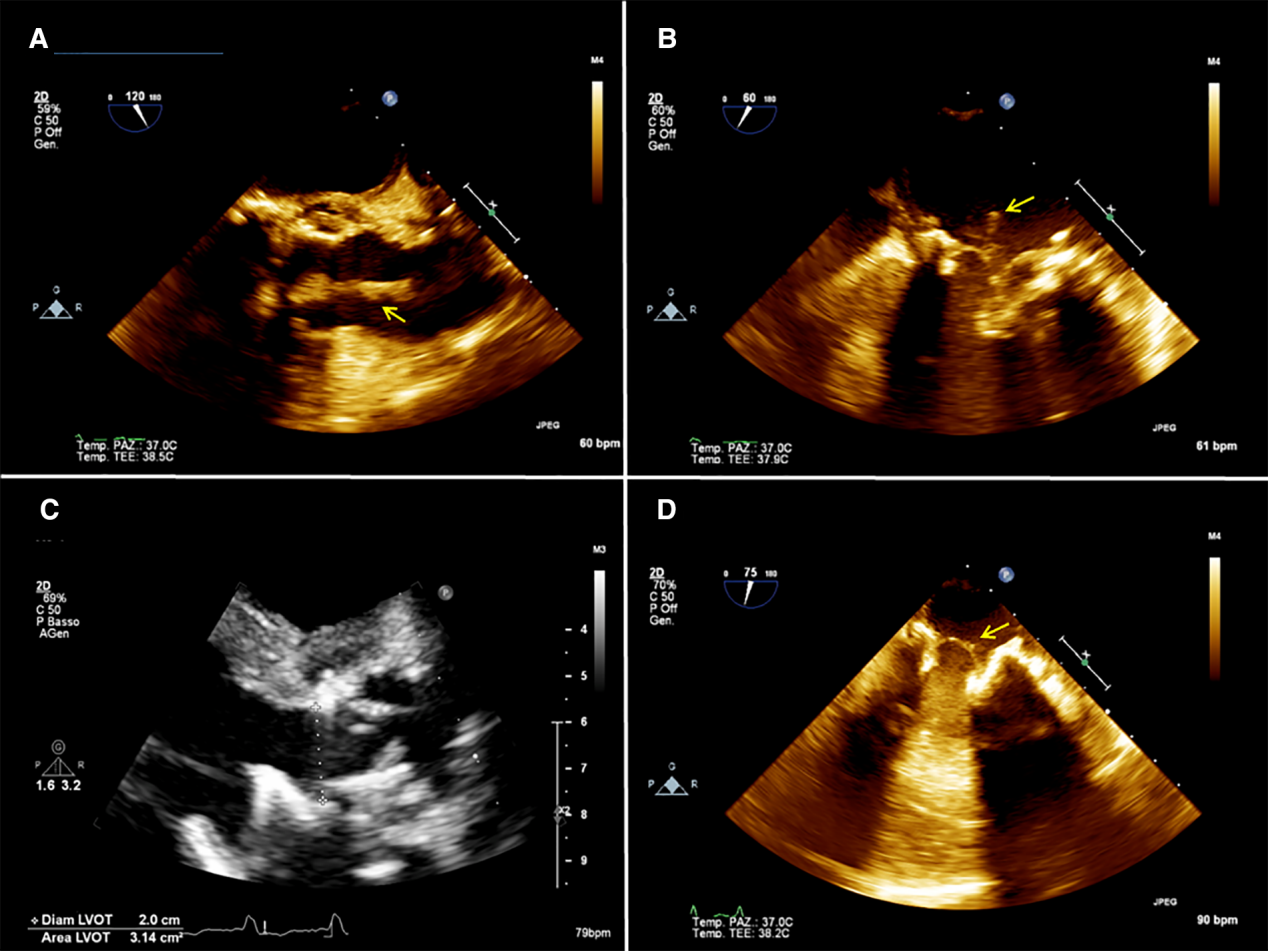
(A) 2D preoperative transesophageal echocardiography (TEE) showing the presence of a mass (arrow) attached to the aortic prosthesis and floating in the aortic root. (B) 2D preoperative TEE showing a mass (arrow) attached to the mitral prosthesis on the atrial side. (C) postoperative 2D TEE showing no residual mass on the aortic prosthesis. (D) postoperative 2D TEE showing a residual minimal stump (arrow) on the mitral prosthesis.
